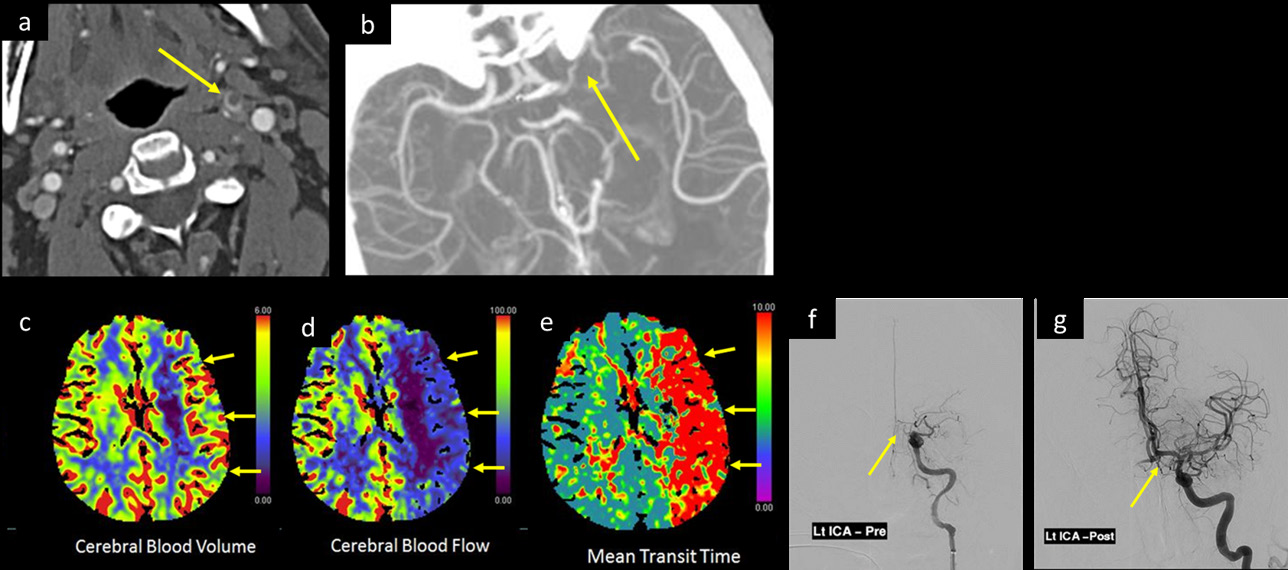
A 30-year-old male was tested positive for COVID-19 with marked deterioration of respiratory symptoms, suddenly developed aphasia and right-side-weakness with a low GCS. CTA of the head and neck, axial images at (a) suprahyoid neck and (b) at Sylvain fissure levels, show a floating thrombus within the cervical left ICA with intracranial extension occluding the left ICA terminus and M1 segment of left MCA (arrow in a and b). CTP of the brain post-processed (c) CBV image, (d) CBF, and (e) MTT color map images display large area of mismatched defect, with large perfusion defect along the left MCA territory showing decreased CBF, prolonged MTT, and compensated CBV (short arrows in c, d, and e respectively). Catheter angiography (f) before and (g) after successful thrombectomy with aspiration of the clot from left ICA show recanalization of left MCA and ACA. Unfortunately, few days later, the left ICA reoccluded. CBF, cerebral blood flow; CBV, cerebral bloodvolume; CTA, CT angiogram; CTP, CT perfusion; GCS, Glasgow coma scale; ICA, internalcarotid artery; MTT, mean transit time.
